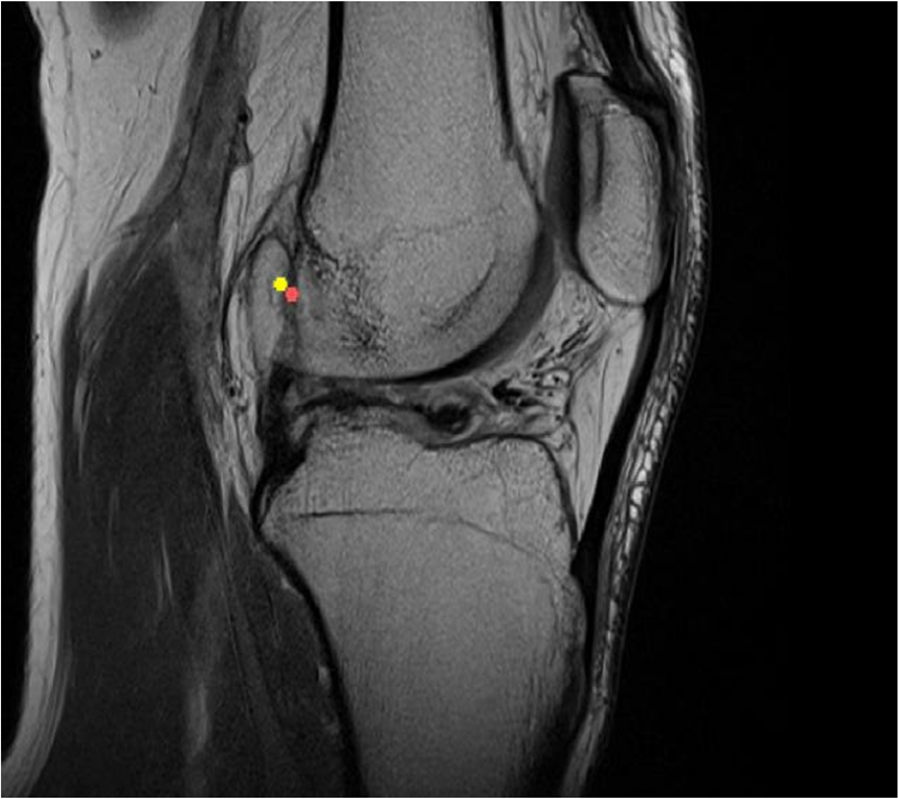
Example of the intraobserver agreement. Figure showing a sagittal slice of an MRI with two measurements from the same observer with at least one week interval
